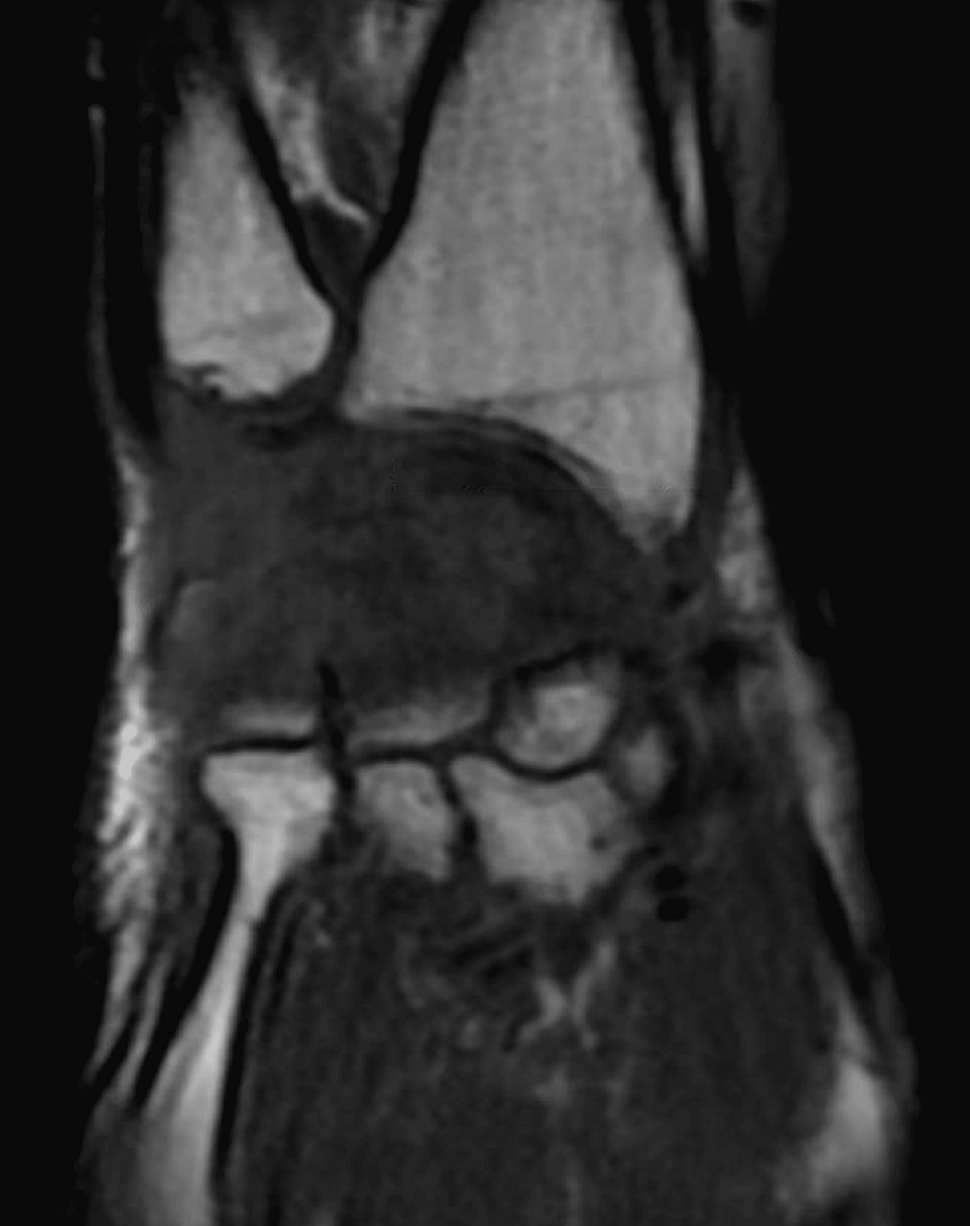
Coronal T1 non-fat suppressed sequence MRI. The right wrist demonstrates significant bone marrow edema throughout the scaphoid, lunate, majority of the capitate, and hamate with multifocal osseous erosions within the proximal and distal pole of the scaphoid. Findings concerning osteomyelitis and recommend follow-up with a bone biopsy.
